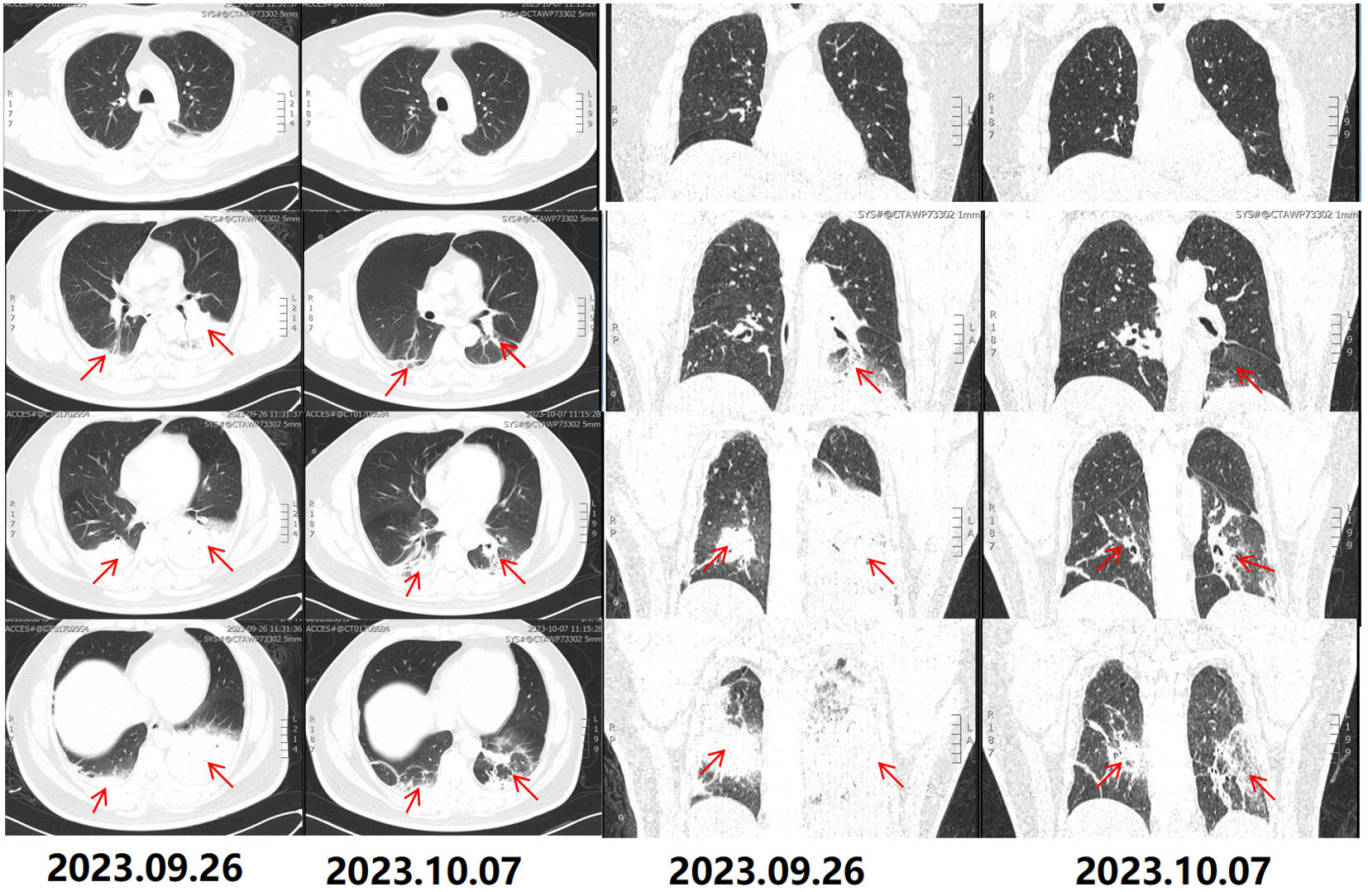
CT scans upon hospital admission. The chest CT on September 26, 2023, reveals bilateral lower lobe consolidation, suggestive of infection, with multiple small and slightly enlarged lymph nodes in the mediastinum. Follow-up lung CT on October 7, 2023, shows partial resolution of the bilateral lower lobe consolidation, indicating improvement of the infection, with the mediastinal lymph nodes unchanged. The red arrows indicate lung consolidation.
